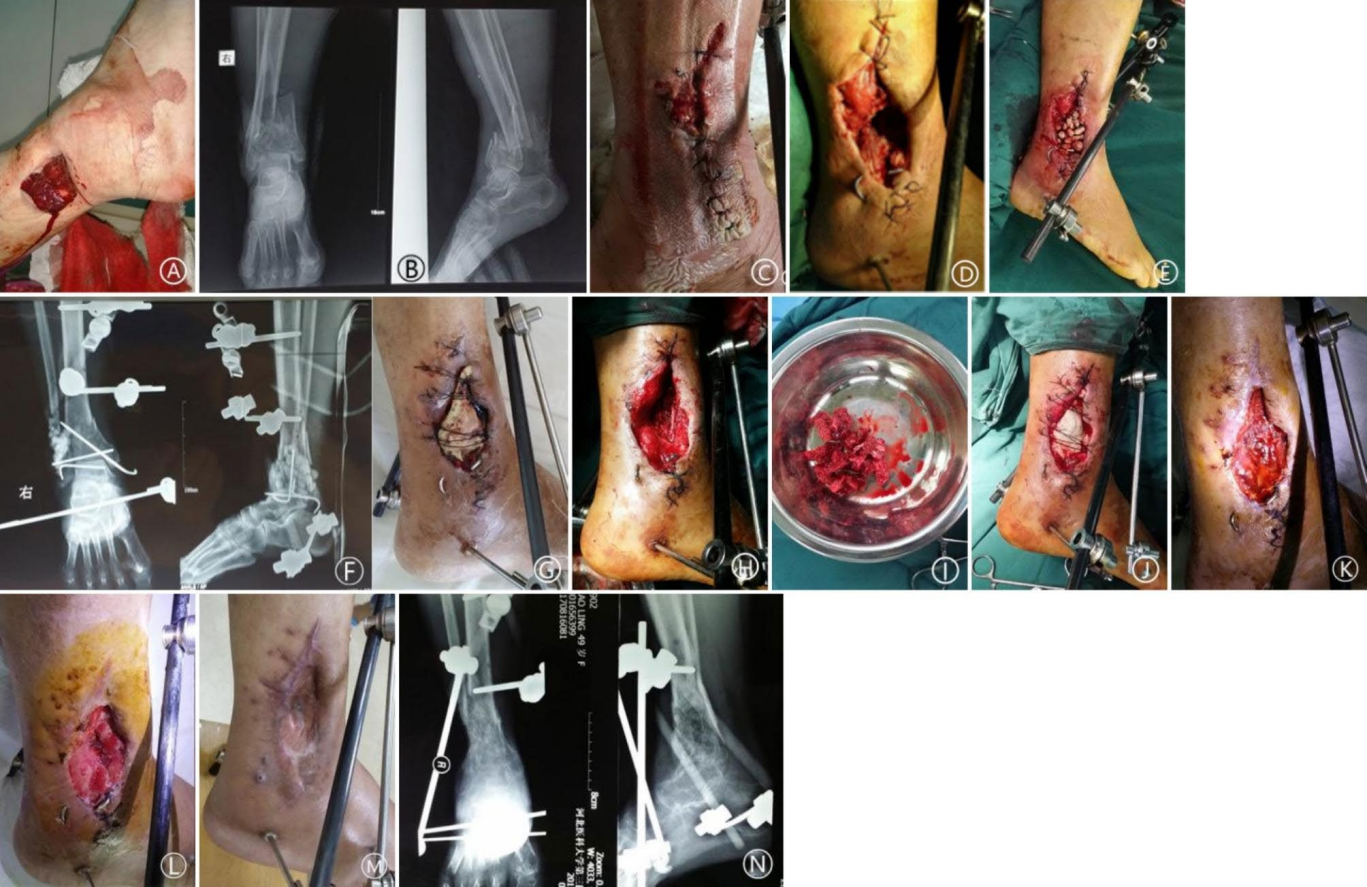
A: a 48-year-old woman presented with open fracture in the right distal tibia and 6 × 5 cm skin loss.B: X-ray. C: emergency debridement and VSD drainage. D: removal of the infected fibula and surrounding soft tissue. E: filling of the osseous cavity with antibiotic-containing cement beads. F: postoperative X-ray. G: the wound after 2 weeks of treatment. H: fresh granulation tissue upon removal of the bone cement beads. I/J: autologous bone grafting using iliac bone, followed by coverage with bone cement sheet. K/L: granulation tissue over the bone graft upon removal of the bone cement sheet 2 and 3 weeks later, respectively. M: 2 months after skin grafting. N: X-ray at 3.5 months after the bone grafting
